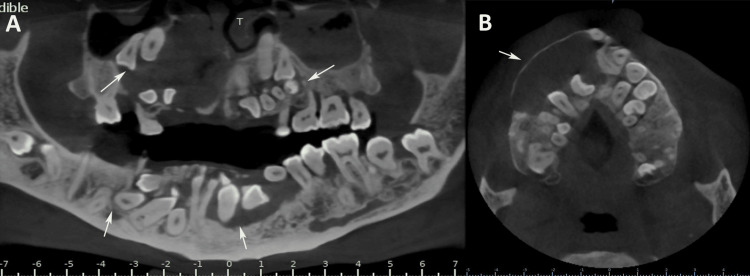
CBCT findings (A) Panoramic CBCT reconstruction revealing approximately 45 impacted teeth with cystic changes in the mandible and proximity of root apices to the inferior mandibular border. (B) Axial CBCT sections showing a large dentigerous cyst in the right maxilla involving teeth from the right upper central incisor to the first molar, with cortical plate breach and nasal cavity involvement CBCT: cone beam computed tomography
